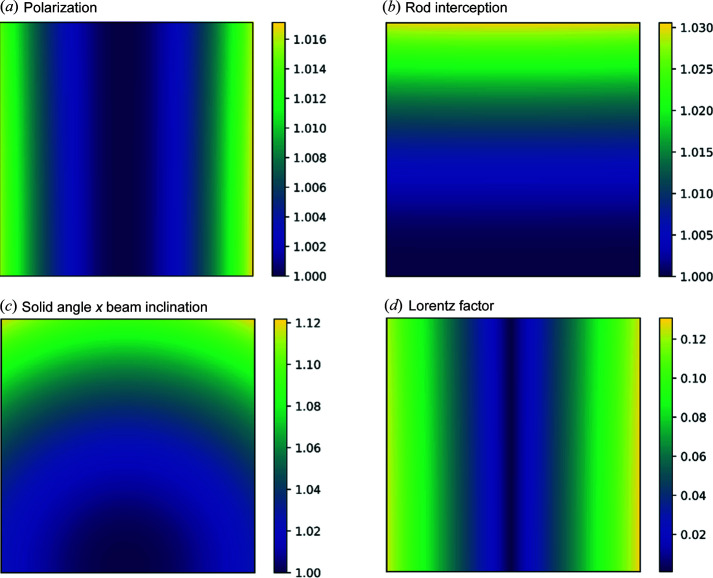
Reciprocal intensity correction factors. Calculated for a 2048 × 2048 pixel (200 × 200 µm) detector at a distance 1.6 m from the sample with an incidence X-ray energy of 73.7 keV which corresponds to the same parameters as the experimental data shown in Figs. 4 ▸ and 6 ▸. The effect of individual correction factors is shown separately and not combined except where indicated.
